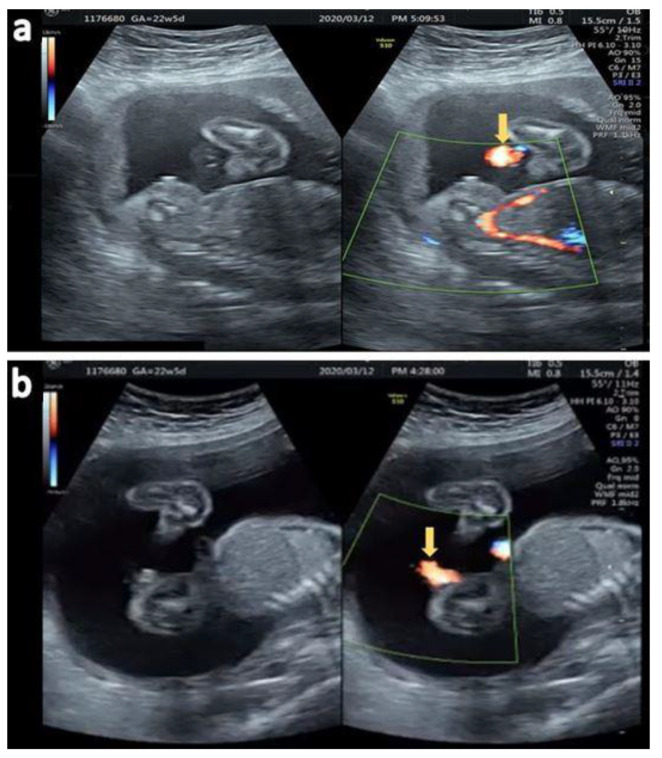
(a) Fanning of the urinary stream jet proximal to the perineum (arrow) (b) Sagittal view of the ventral jet of urinary stream under color Doppler (arrow).
